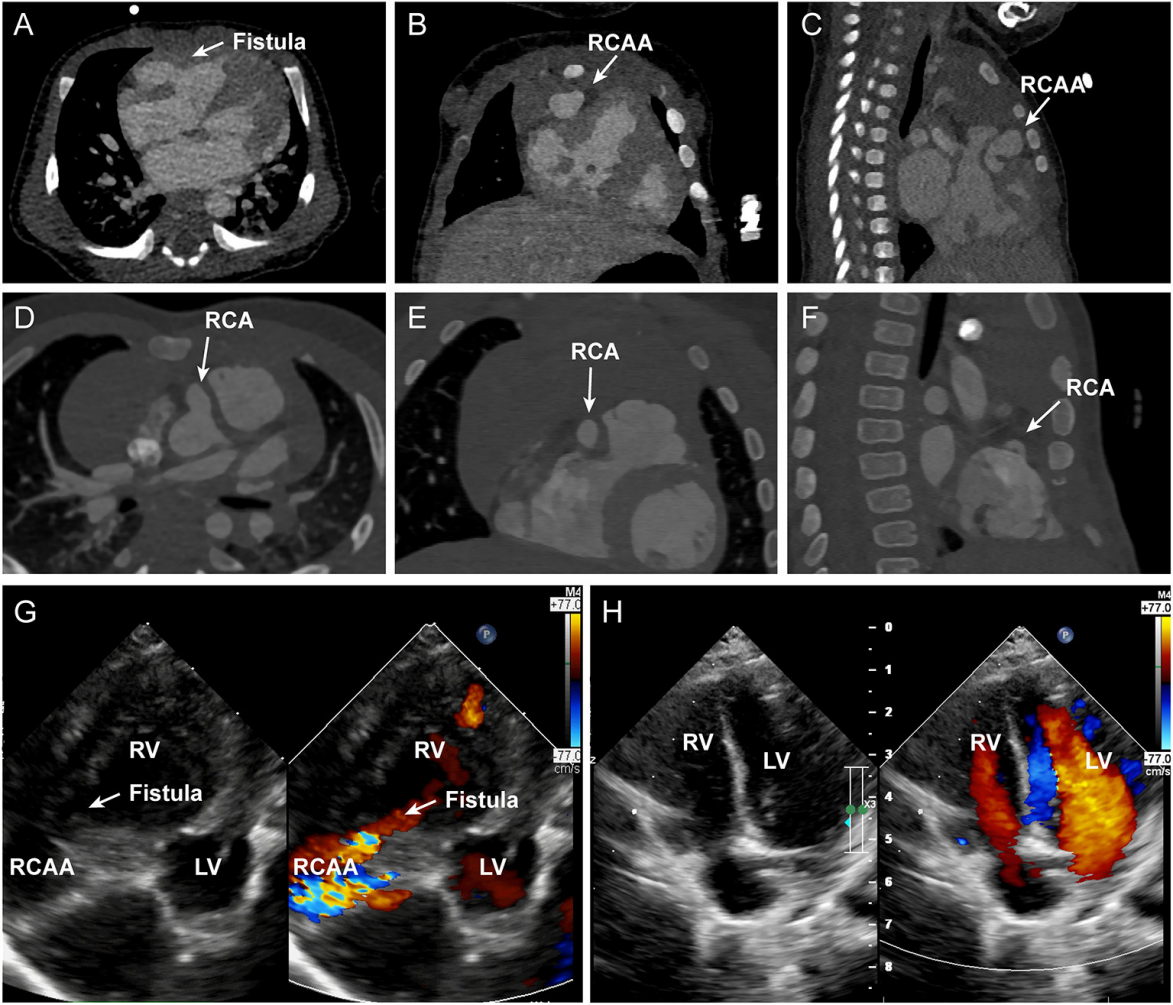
(A–C) depict the axial, coronal, and sagittal panel of the preoperative CTA, respectively. The white arrows indicated the fistula and RCAA. (D–F) depict the axial, coronal, and sagittal panel of the 6-months follow-up CTA, respectively. The white arrows indicate the mild dilated RCA. (G,H) show the preoperative and postoperative echocardiography images of the patient. A large fistula and RCCA to RV shunt was indicated by the arrow. RCA, right coronary artery; RCAA, right coronary artery aneurysm; LV, left ventricle; RV, right ventricle.
